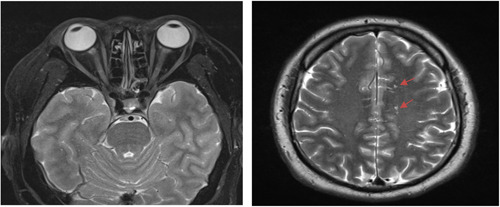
T2 weighted MRI of the brain and optic nerve showing no optic nerve enhancement. There are multifocal hyperintense white matter lesions at the subcortical regions of bilateral frontal lobes, left parietal lobe and left posterior cingulate gyrus (orange arrows).
